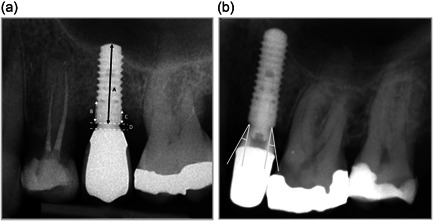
(a) Radiograph of an implant with marginal bone level measurements. A: length of the implant, B + C: distance mesial (B) and distal (C) from the implant shoulder to the first bone‐to‐implant contact, and D: abutment height. (b) Radiograph showing measurements of the emergence angle relative to the longitudinal axis of the implant
